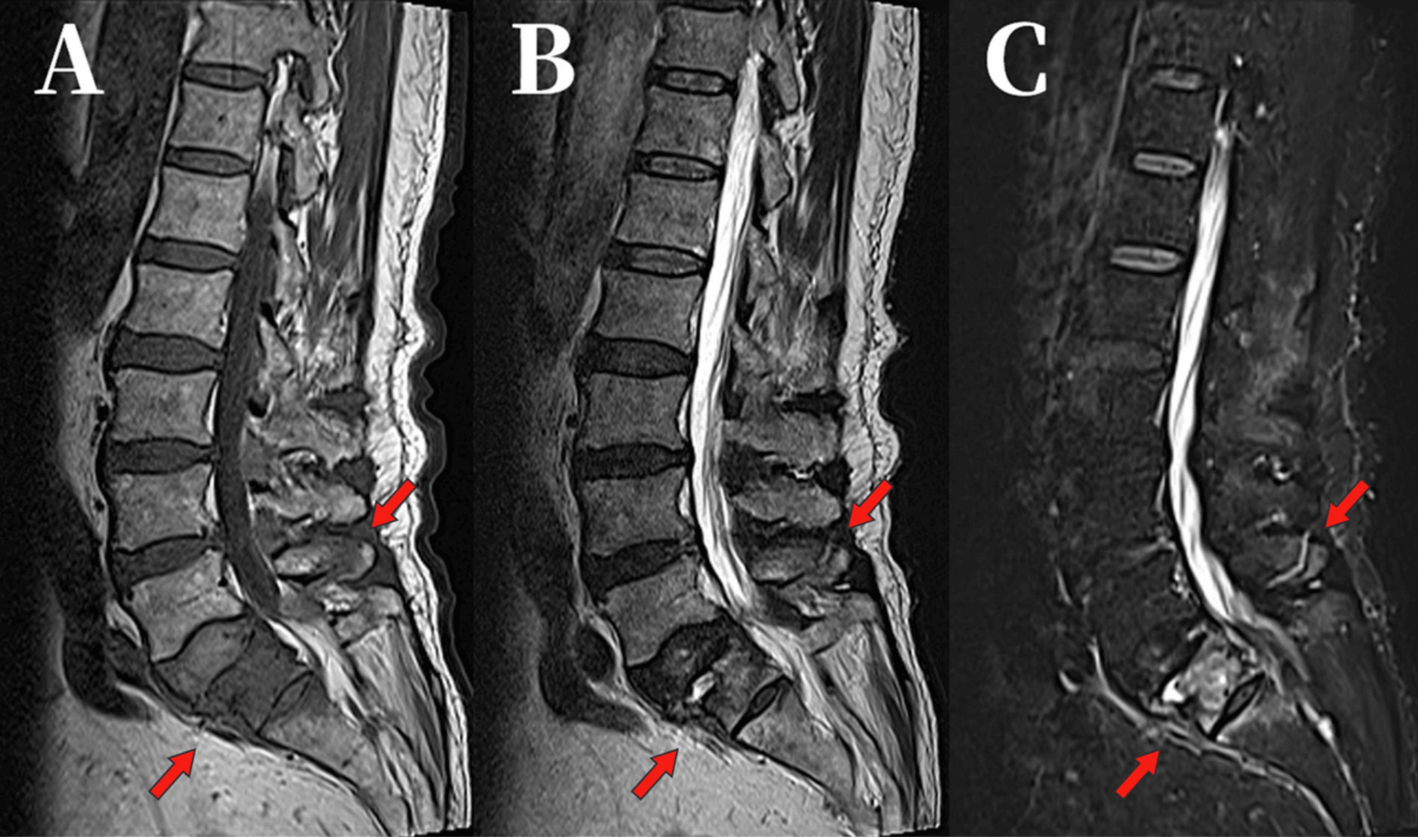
Sagittal MRI images. (A) T1-weighted (B) T2-weighted (C) STIR images showing diffuse marrow edema in S1 and an L5 spinous process fracture (4 weeks after injury). MRI: magnetic resonance imaging, STIR: short tau inversion recovery.
